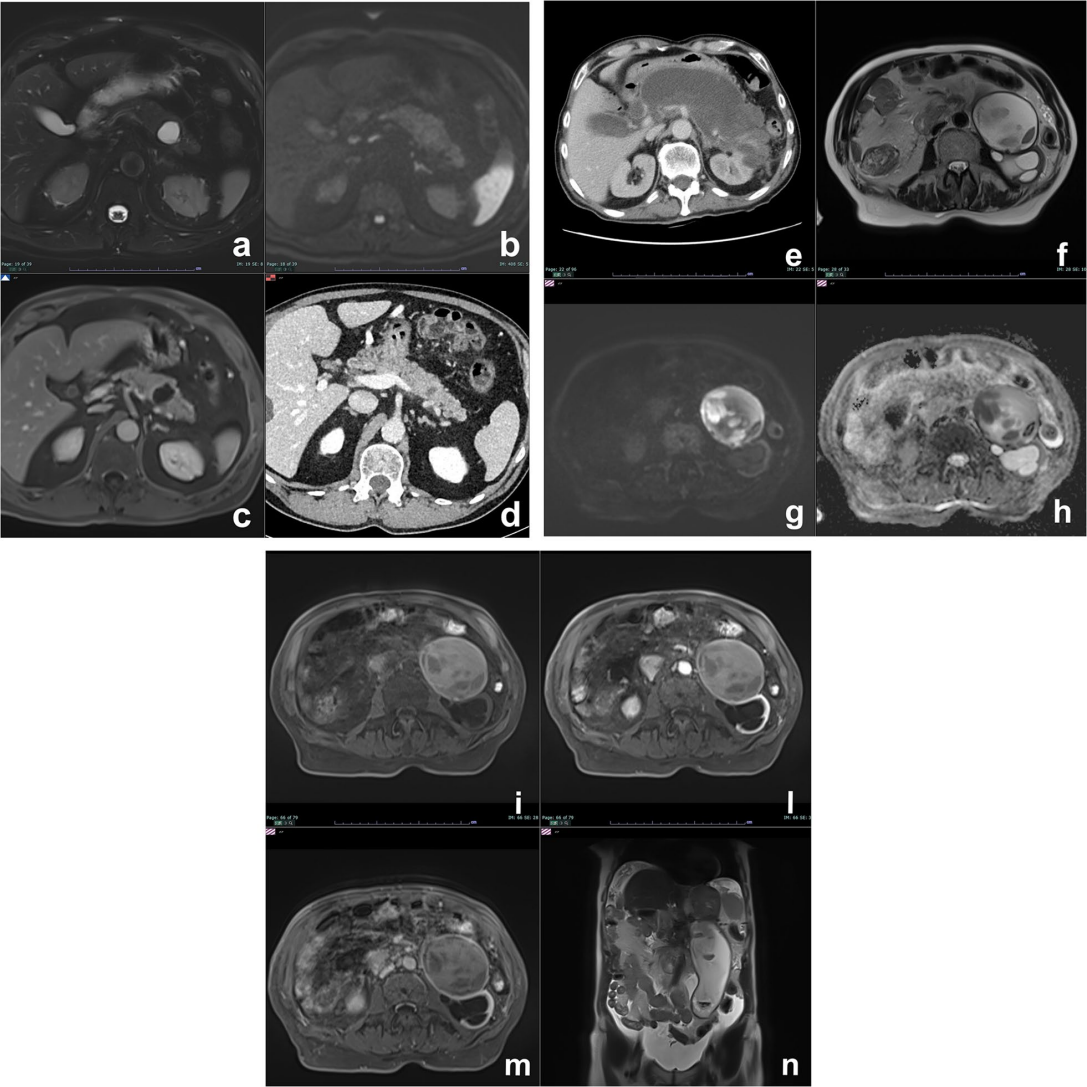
Cystic complications of acute pancreatitis. a–d Pseudocyst. Patient with a previous interstitial edematous pancreatitis. At follow-up after 1 year, a cystic lesion with no septa or debris is appreciable in the tail of the pancreas, hyperintense on T2w (a), without high signal intensity on high b value DWI (b) and no enhancement after contrast media administration (c). At further follow-up after 6 months, the lesion is decreased in size (d). e–n: WON. A large necrotic collection is appreciable in a patient with necrotizing acute pancreatitis (e). After 1 year, a large collection with debris is still appreciable in the tail of the pancreas, clearly visible on T2w as cystic lesion with low signal intensity foci (f). Debris show inhomogeneous signal intensity on DWI (g), ADC map (h) and T1 unenhanced (i), but do not show any enhancement after contrast media administration (l, m). On T2 HASTE coronal view, the caudal extension of the collection is appreciable (n)
